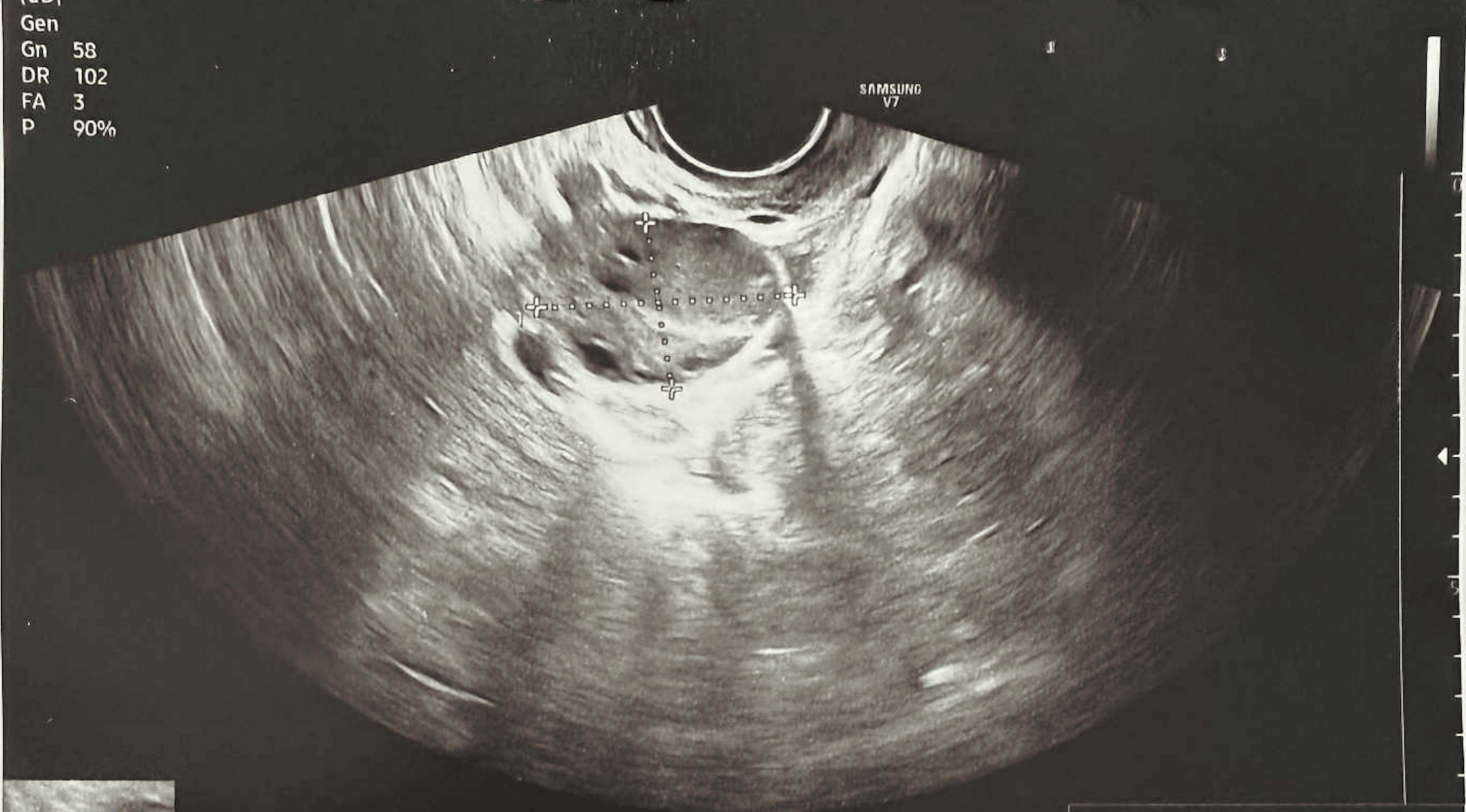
Ultrasound image from the day of patient admission showing a heterogeneous hyperechoic area
